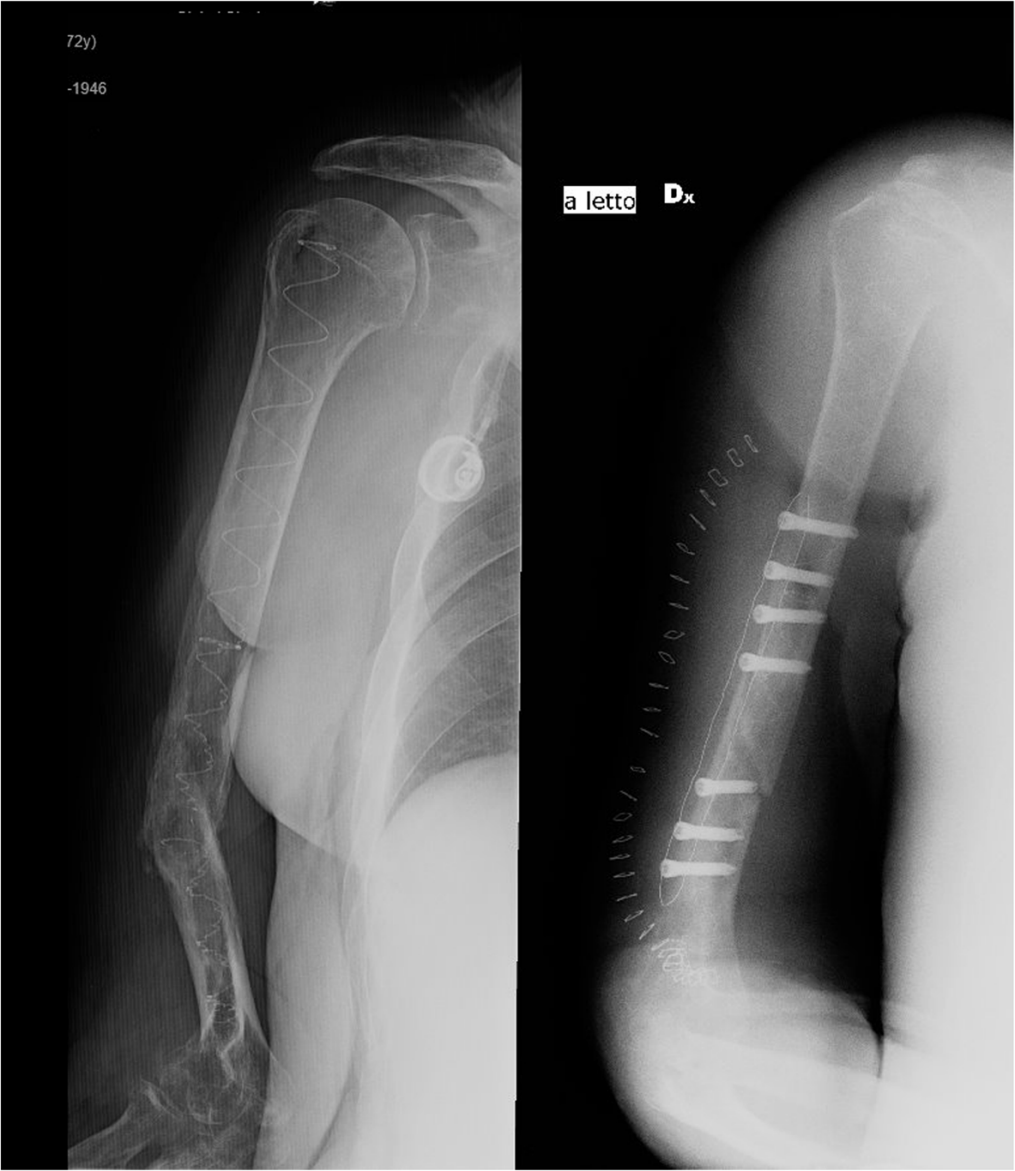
X-ray showing the rupture of the IS-PBSS on the left and the postoperative control after open reduction and plating; considering the poor bone quality, the IS-PBSS was maintained inside the canal as an augmentation to increase the screws’ effectiveness
